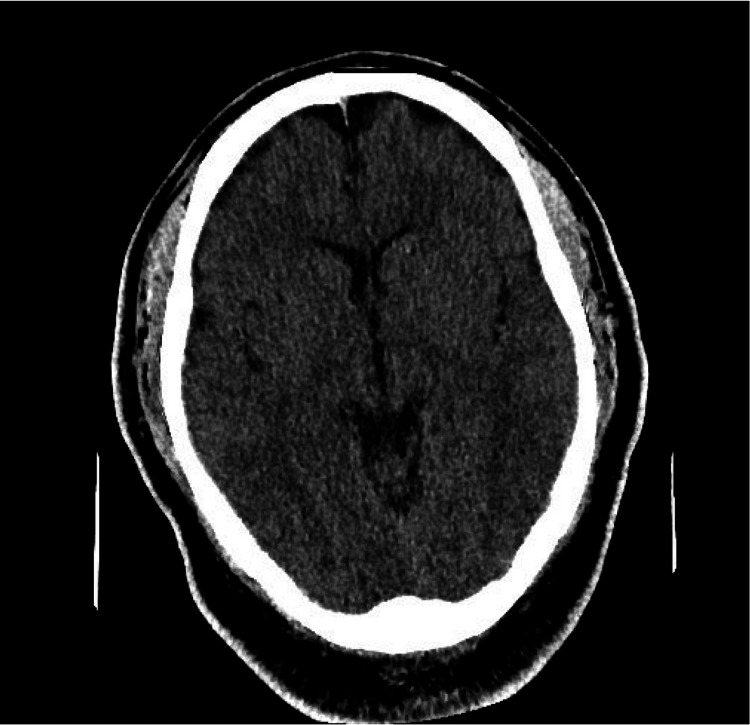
CT of the head with unremarkable findings
